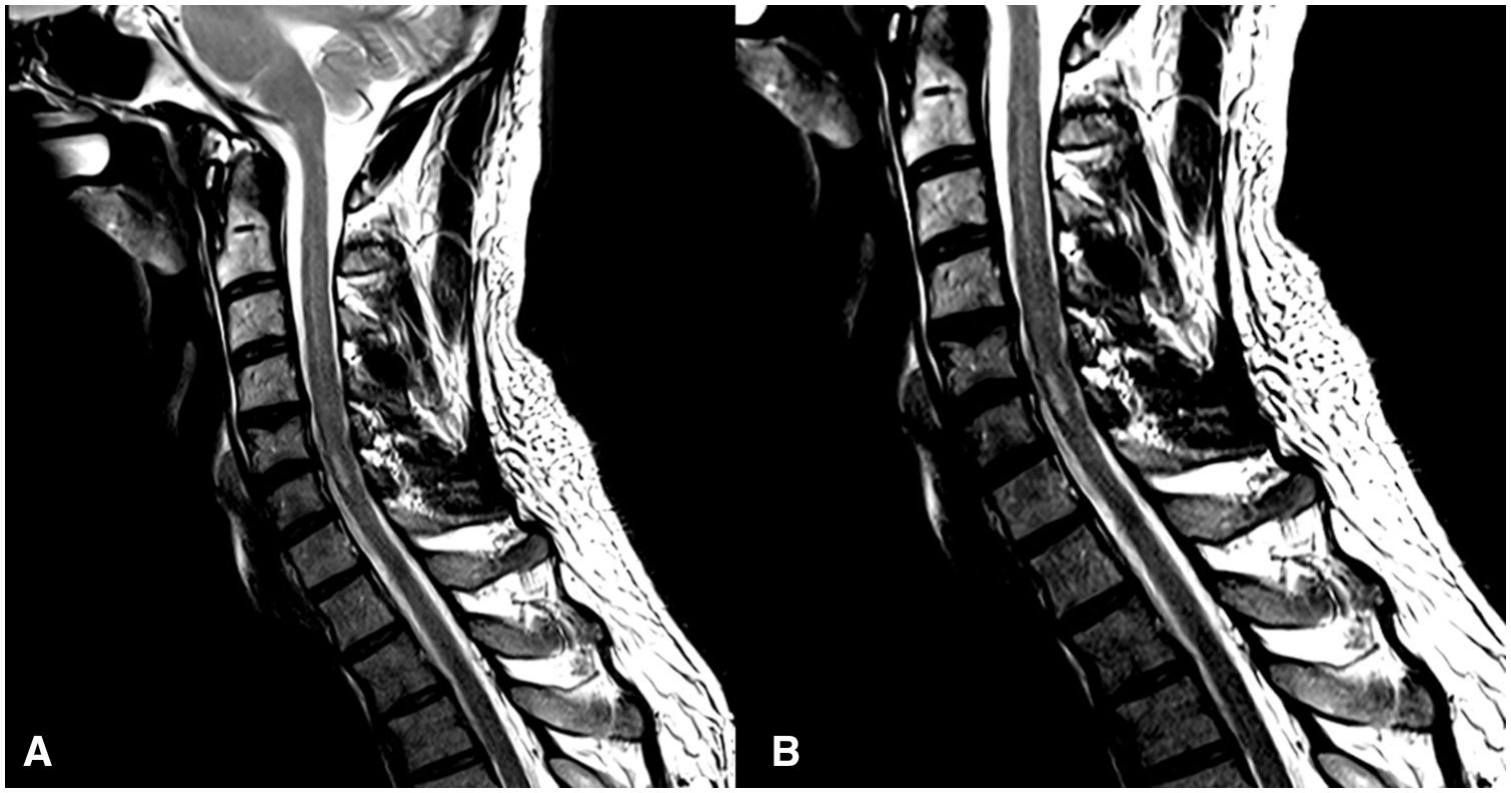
(A and B) Sagittal T2-weighted MRI cervical spine demonstrating FLAIR hyperintensity reflecting the C5-6 SCI.
